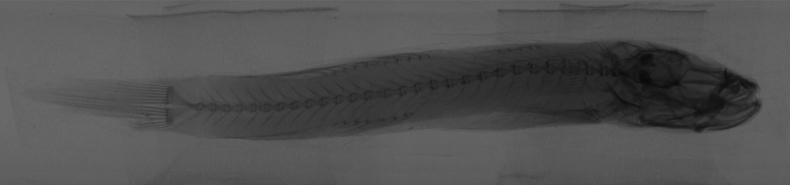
The skeletal system of *R.sudoccidentalis* sp. nov. Radiograph graphs of the whole body for paratype RS20230102, male.
